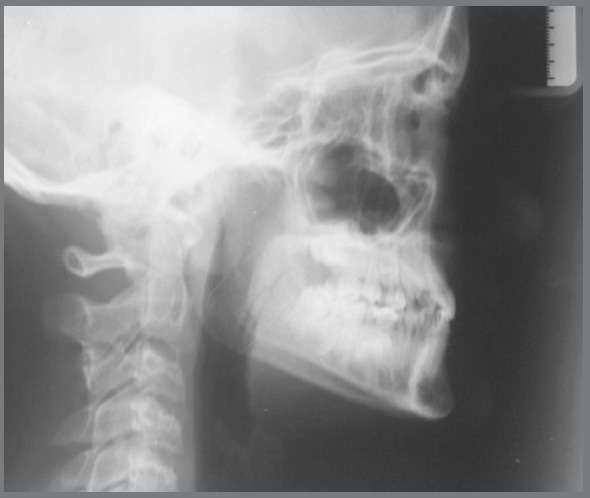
Pretreatment lateral radiograph.
